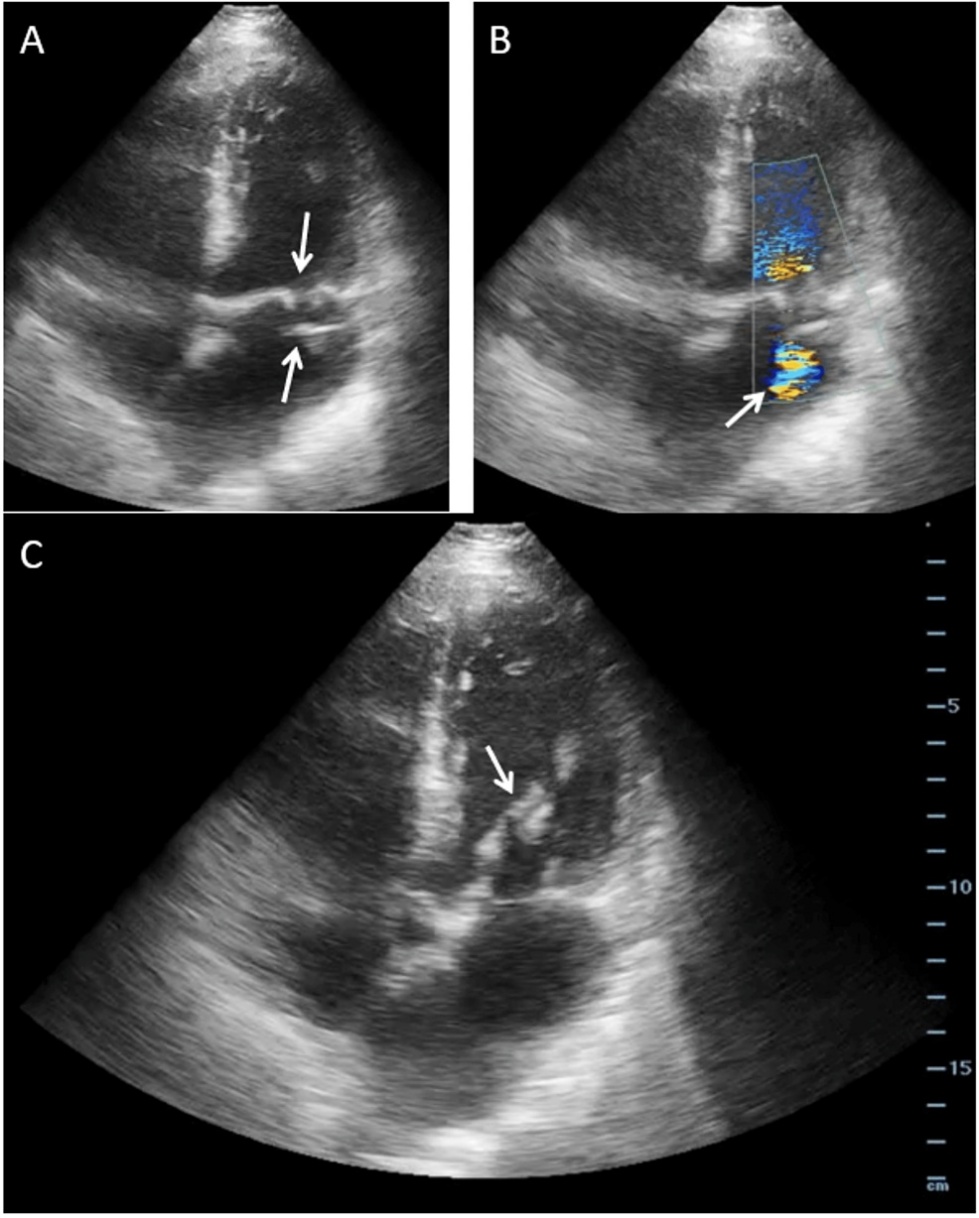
Bedside transthoracic echocardiography Bedside transthoracic echocardiography (apical 4-chamber view) performed by the emergency department (ED) resident physician demonstrating (A) retrograde movement of both mitral valve leaflets into the left atrium (downward arrow) with mass adherent to the anterior leaflet (upward arrow), (B) regurgitant jet across the mitral valve with color flow doppler (arrow), and (C) mobile mass adherent to the anterior mitral valve leaflet (arrow).
